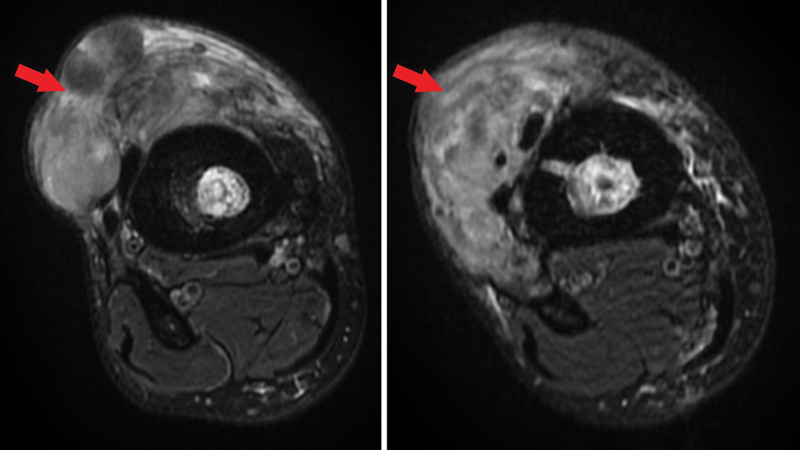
MRI revealed a large, heterogeneous (red arrow), lobulated mass anterior to the mid/distal third tibia likely an inflammatory/infective phlegmon, however, biopsy suggested to rule out neoplastic changes.
